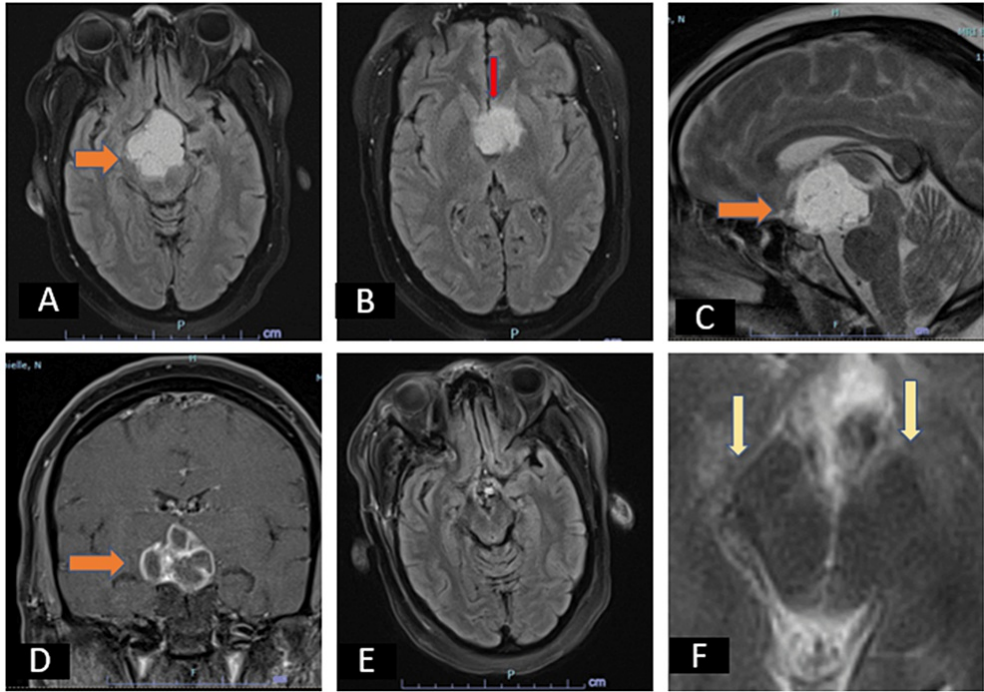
Pre and postoperative brain MRI (T2/SWI) A: T2 Flair axial sequence of preoperative brain MRI showing a craniopharyngioma compressing the midbrain. B: T2 Flair axial sequence of preoperative brain MRI showing a craniopharyngioma compressing the caudates. C: T2 Sagittal T2 postcontrast sequence of preoperative brain MRI showing a craniopharyngioma compressing the midbrain. D: T1 Coronal postcontrast sequence of preoperative brain MRI showing a craniopharyngioma compressing the midbrain. E-F: Postoperative Brain MRI showing loss of normal swallow tail in T2/SWI (F, yellow arrows), with expected surgical changes in brain MRI and no further compression in midbrain (E) MRI: Magnetic resonance imaging; T2/SWI: T2/susceptibility-weighted imaging
